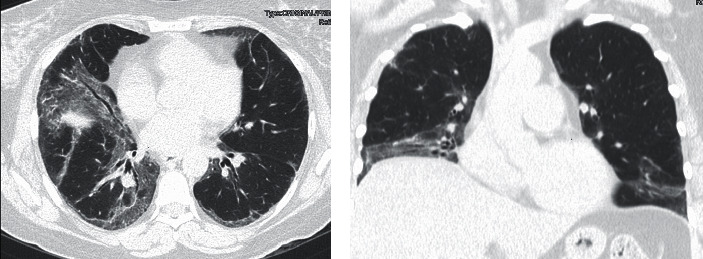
High-resolution computed tomography of the chest (Case 1). (a) Confluent ground glass opacity and consolidation, mainly in the mid and lower lungs, associated with moderate traction bronchiectasis. The distribution is partially subpleural with extension along the bronchial vascular bundles. Lung volumes are markedly reduced. (b) Coronal view demonstrating peri-diaphragmatic consolidation with an abrupt transition to normal parenchyma.
